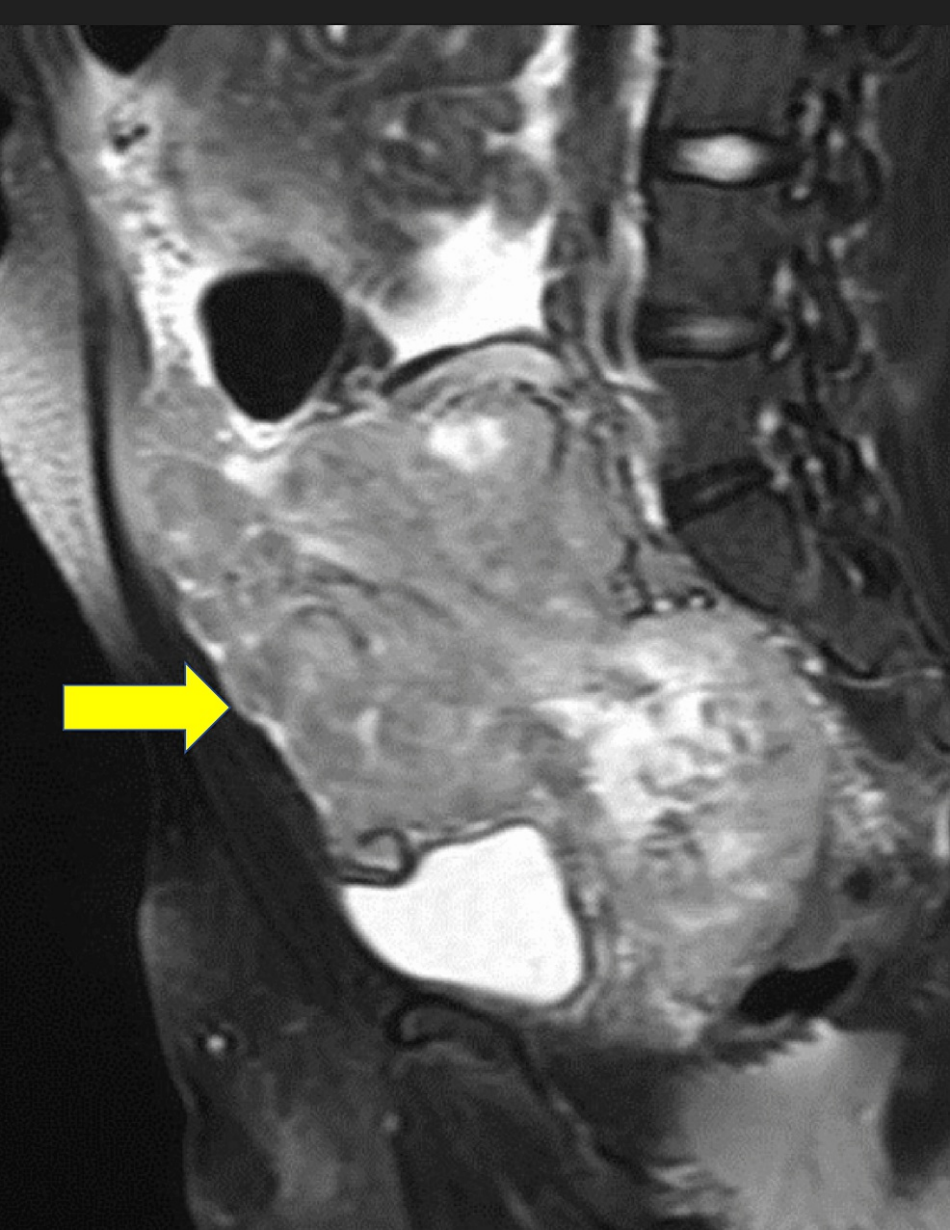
Sagittal T2 MRI demonstrating a pelvic mass (yellow arrow). MRI: magnetic resonance imaging
